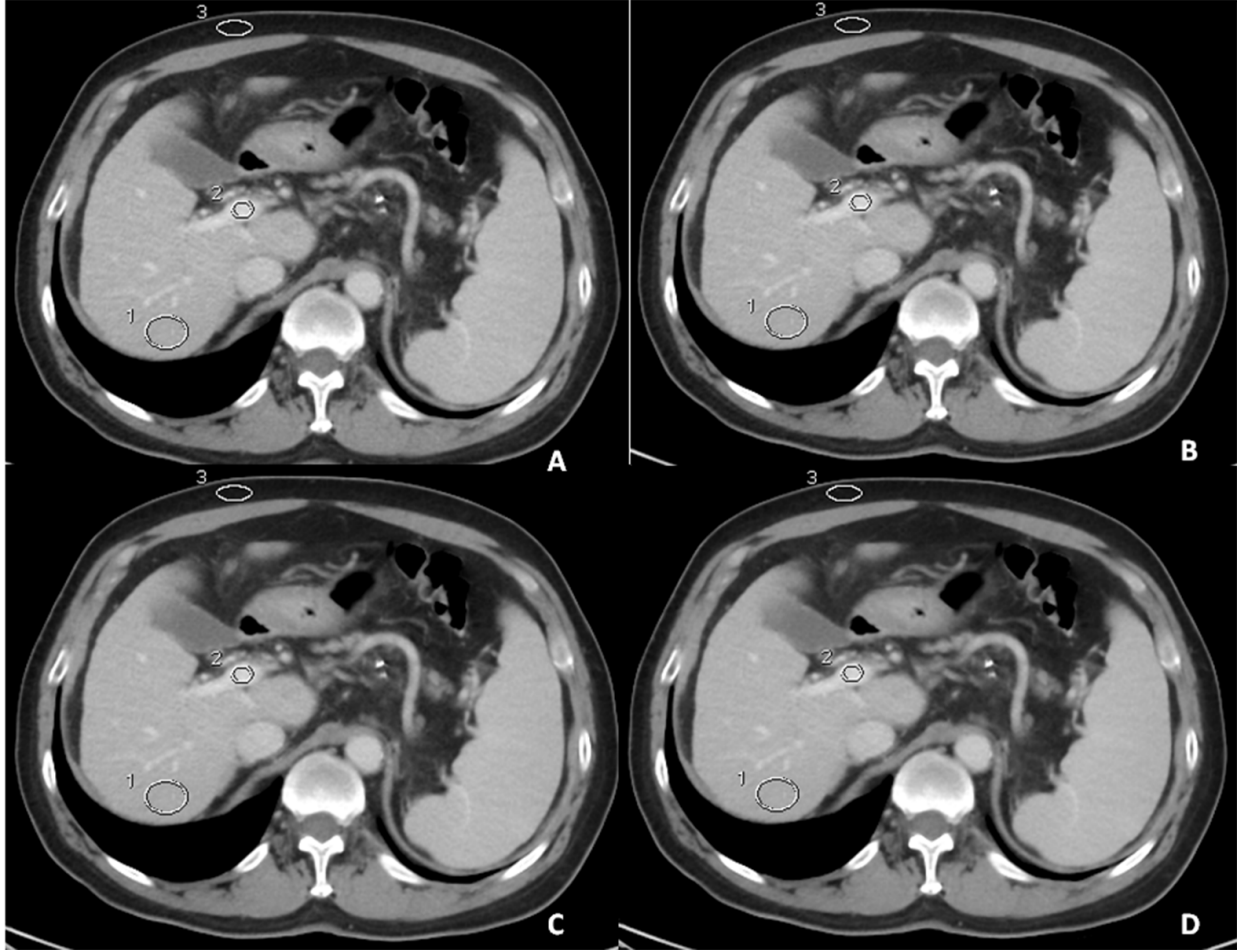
Enhanced axial images at portal venous phase of a 63-year female patient with portal hypertension secondary to liver cirrhosis with different percentage ASIRs, for objective evaluation of CT portal venography quality. (A), (B), (C) and (D) were obtained with 0%, 30%, 50%, and 70% percentage ASIR reconstruction, respectively. The marked regions were ROIs in the main stem of portal vein, liver parenchyma, and subcutaneous fat.
